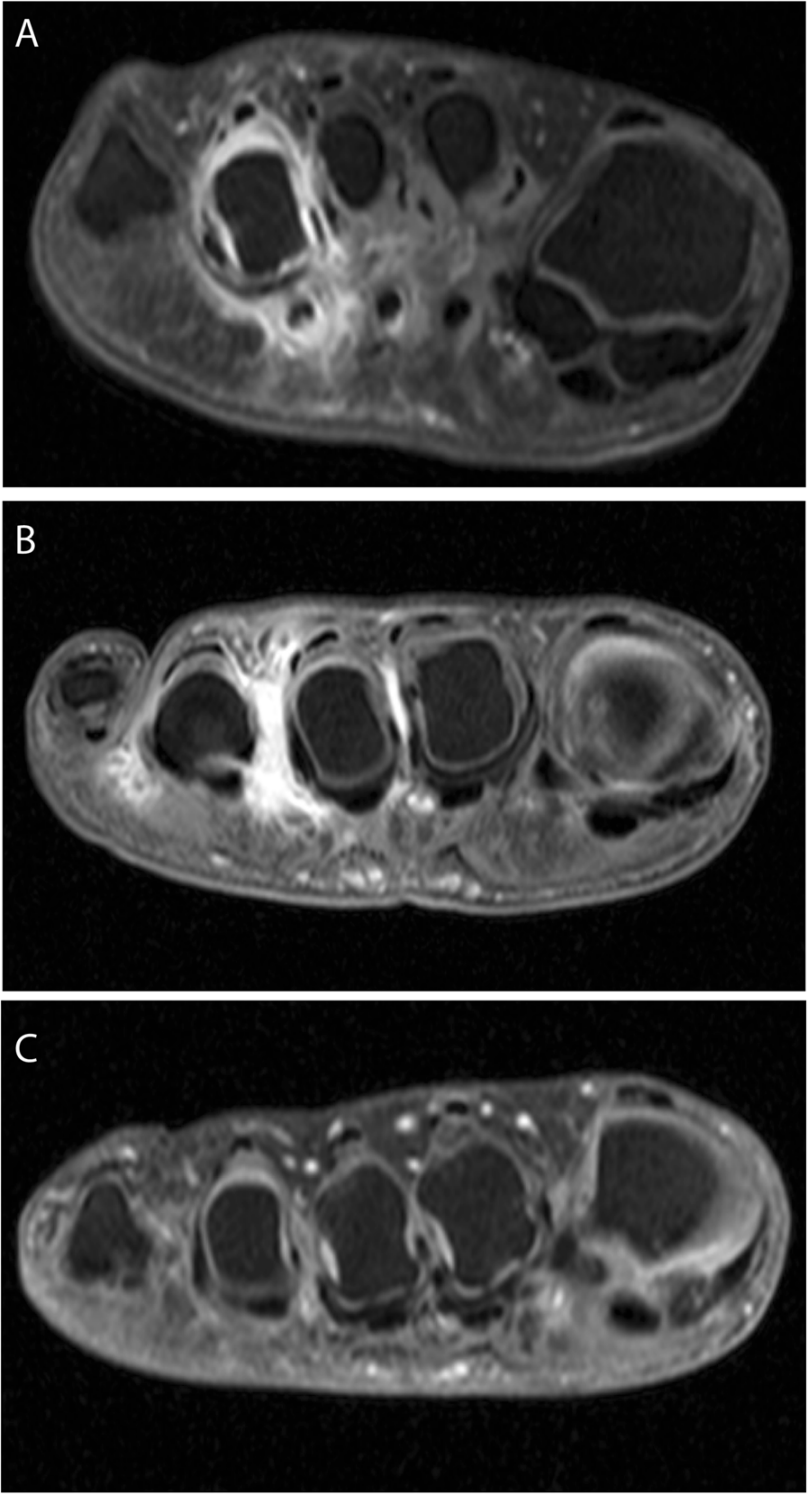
MRI examples of MTP joints that are swollen upon physical joint examination (PE) with MRI-detected inflammation (a), with intermetatarsal bursitis (b), and without any signs on MRI (c). a A patient with a swollen MTP 4 upon PE and with MRI-detected synovitis and tenosynovitis at the same joint. b A patient with swollen MTP 3 and 4: there is no MRI-detected synovitis, tenosynovitis, or osteitis; there is intermetatarsal bursitis at the 2nd, 3rd, and 4th intermetatarsal spaces. c Patient with swollen MTP 2, 3, and 4 upon PE but no MRI-detected inflammation or intermetatarsal bursitis on MRI
